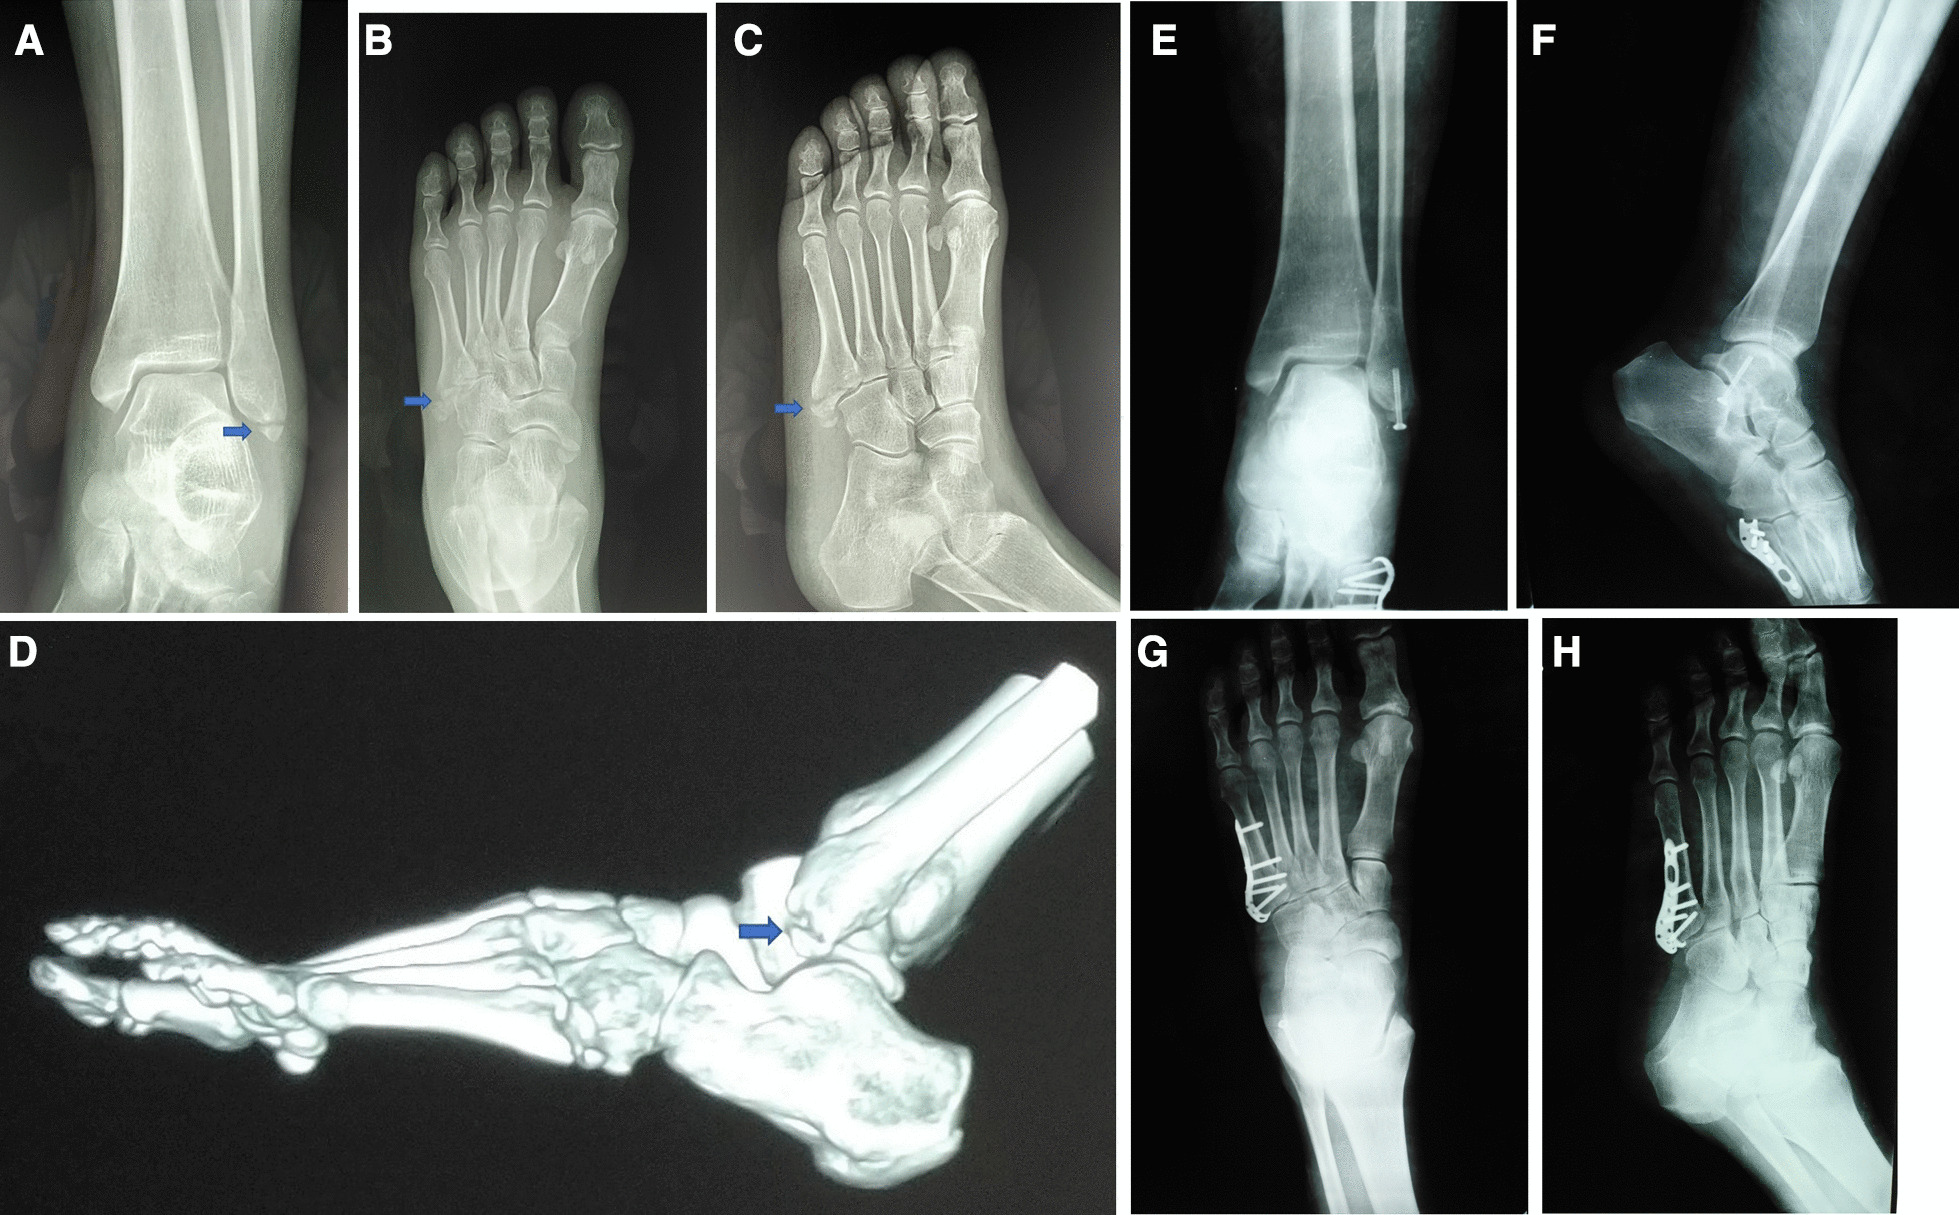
A Ankle anteroposterior /lateral radiographs showed avulsion fracture at the tip of lateral malleolus, arrow showed lateral malleolus fracture. B, C Preoperative foot anteroposterior/oblique radiographs showed displaced-fifth metatarsal base fracture, arrow showed fifth metatarsal base fracture. D Preoperative CT showed lateral malleolus avulsion fracture block and fifth metatarsal base fracture, arrow showed lateral malleolus fracture. E, F Postoperative ankle radiographs showed that lateral malleolus avulsion fracture was fixed with a cannulated screw. G, H Postoperative foot radiographs showed that the fifth metatarsal base fracture was fixed with a hook plate, and the fracture was anatomically reduced
